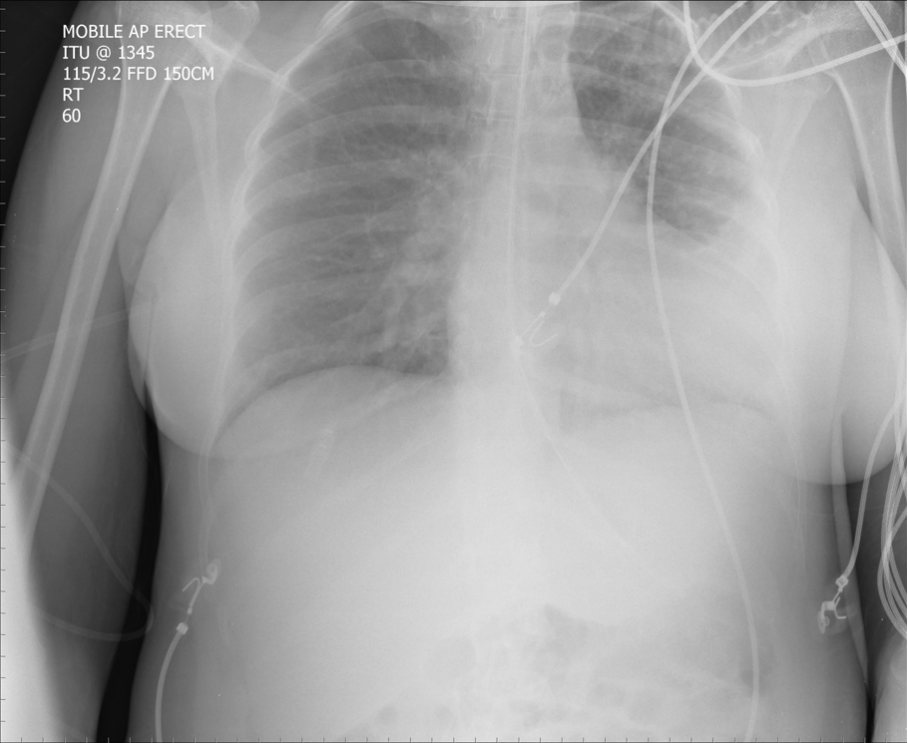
Chest radiograph demonstrating infective infiltrate/consolidation.
